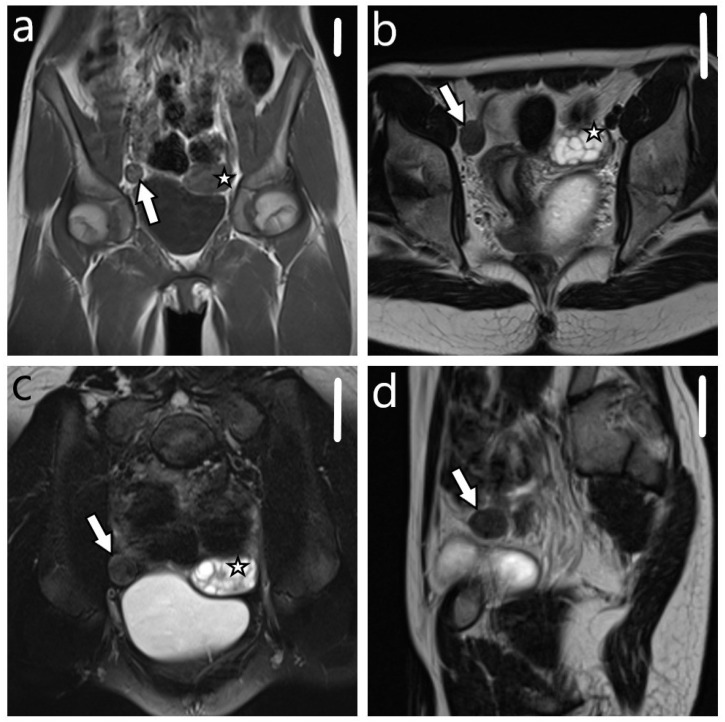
A 12-year-old adolescent who complained of abdominal distention and pelvic discomfort was admitted to the hospital. A magnetic resonance imaging (MRI) scan showed a large right-sided well-circumscribed pelvic solid mass arising in the round ligament measuring 2.5 × 2.0 × 2.0 cm (a–d, arrow; scale bar 5 cm). It has an *isointense* signal on T1WI ((a)—coronal plane), hypointense signal on T2WI ((b–d)—coronal, axial, and sagittal planes, respectively). There was a restricted diffusion on the DWI and ADC map. The initial MRI was interpreted as intraligamentary leiomyoma. The left ovary with multiple follicles was visualized (a–c, asterisk).
